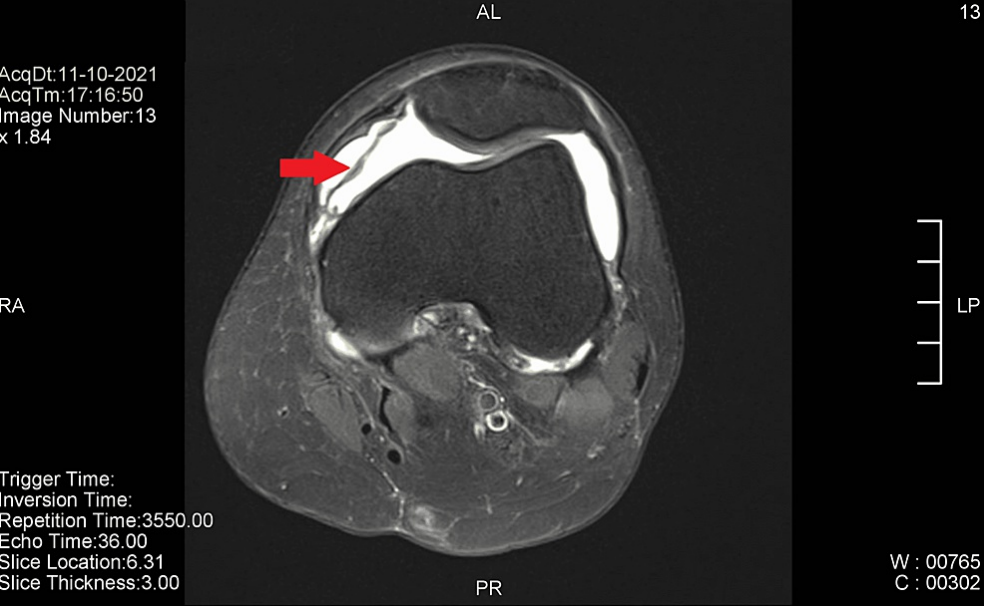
MRI of the knee joint (cross-sectional view). The red arrow points to the tumor.
